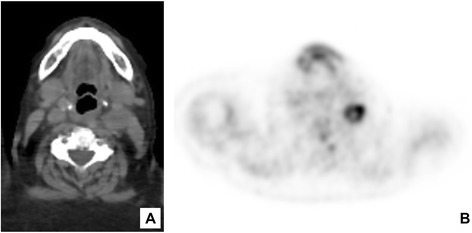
PET-CT: a Axial CT image of the maxilla-facial region showing a left retromandibular mass. b PET image showing tracer hyperaccumulation at the retromandibular and left parapharyngeal regions
